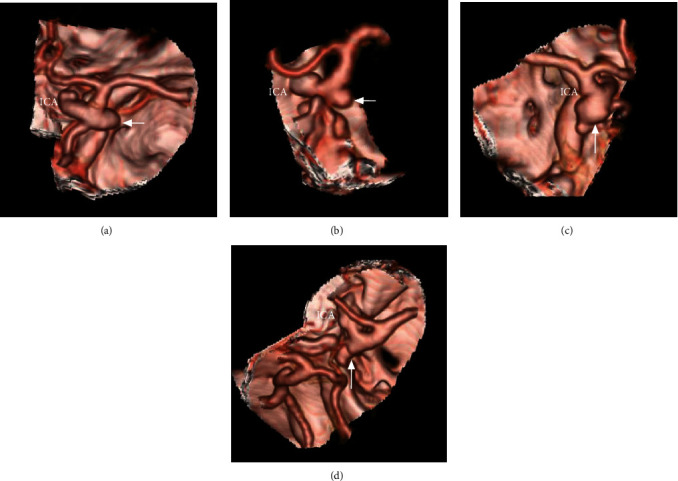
Aneurysm projection in CTA is as folows; (a–b) CTA showing that the aneurysm projected laterally (arrows). (c–d); CTA showing that the aneurysm projected posteriorly (arrows). Abbreviations: CTA: computed tomography angiography; ICA: internal carotid artery.
